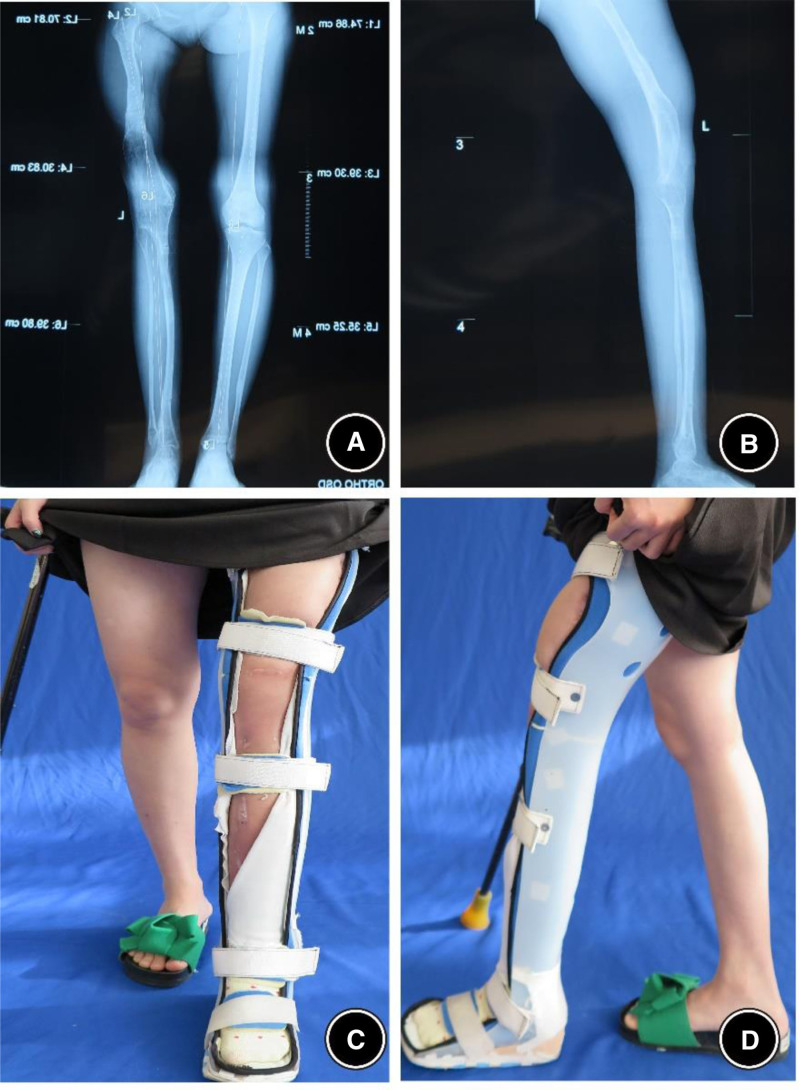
Three years postoperatively, showing significant correction of the fixed knee flexion deformity, with good bone healing in both femoral and tibial lengthening sites, and near-equal length of both lower limbs. (A) Standing anteroposterior radiograph showing near-equal limb. Length and consolidated regenerate at the femoral lengthening site. (B) Standing lateral radiograph. (C) Anteroposterior clinical photograph of the affected limb in standing. (D) Lateral clinical photograph of the affected limb in standing.
